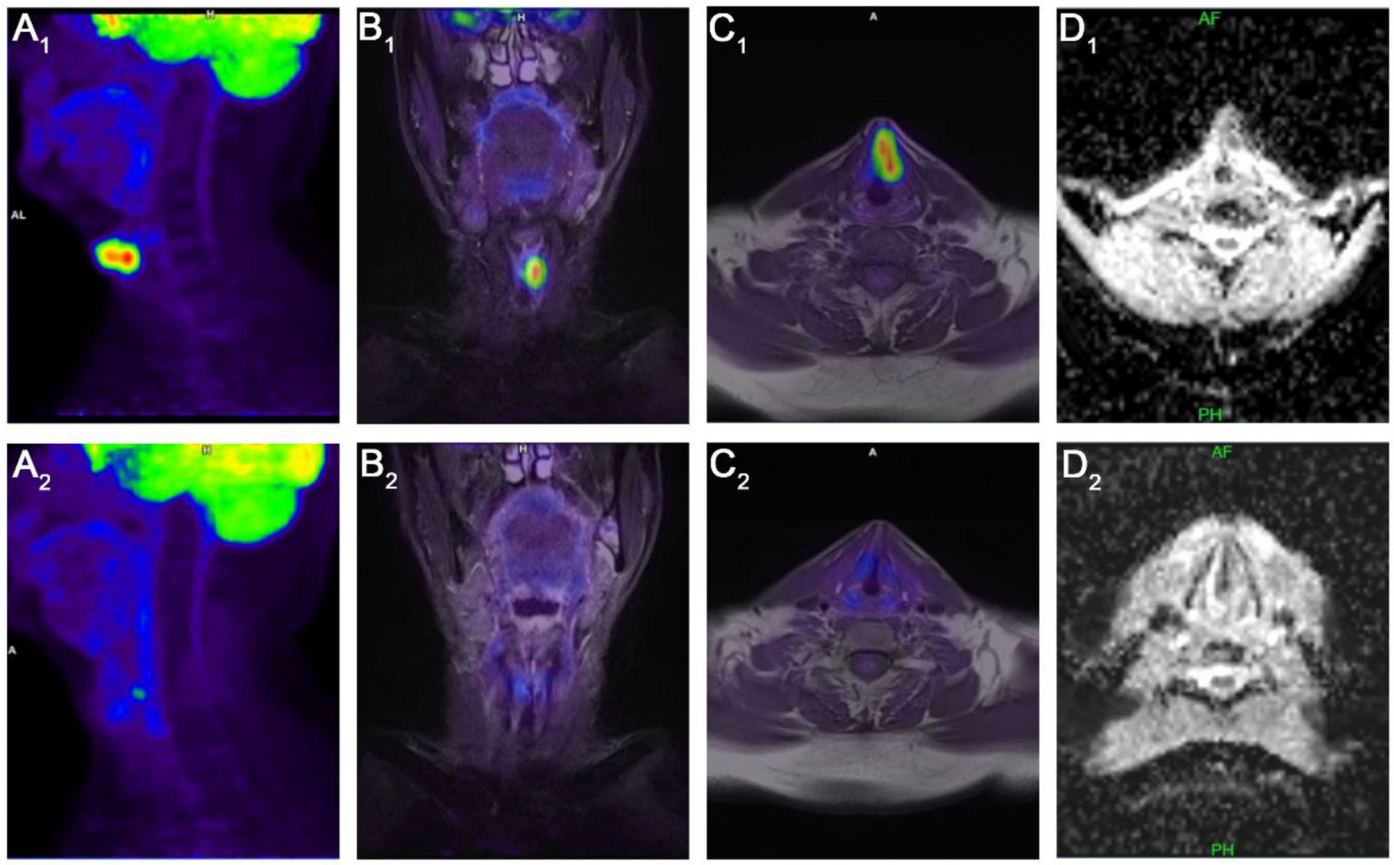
Complete Remission (CR): sagittal PET (A_1_), coronal (B_1_), and axial (C_1_) PET/MR images and axial MR-diffusion weighted imaging (DWI) apparent diffusion coefficient (ADC) map of the tumor (D_1_) show the glottic tumor spread over the supra-, and infraglottic regions, pretreatment maximum of standardized uptake value (SUV_max_): 14.12, peak lean body mass corrected SUV_max_ (SUL_peak_): 8.93, total lesion glycolysis (TLG): 25.46, metabolic tumor volume: 2.97 cm^3^, mean ADC (ADC_mean_): 867.22 + -53.52 × 10^−6^ s/mm^2^. Post-treatment sagittal PET (A_2_), coronal (B_2_), and PET/MR and DWI ADC axial (D_2_) images show complete remission (CR); without any pathologic FDG accumulation, and diffusion restriction on the observed volume.
